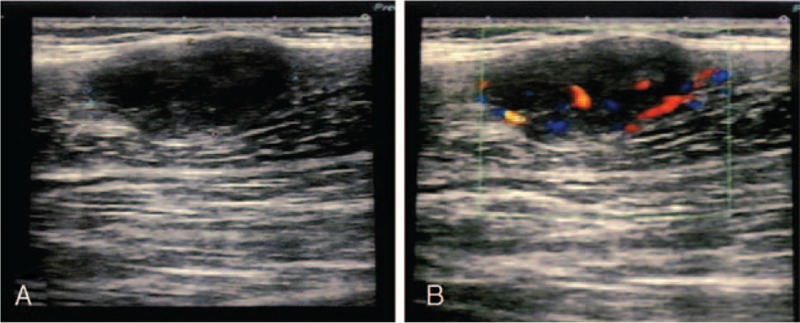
Ultrasonography features of alveolar soft part sarcoma. (A) B-scan ultrasonography revealed a hypoechoic, homogeneous soft tissue mass with clear boundary, which measured 22.7 mm in diameter. (B) Color Doppler flow imaging revealed marked increased vascularity within the mass.
